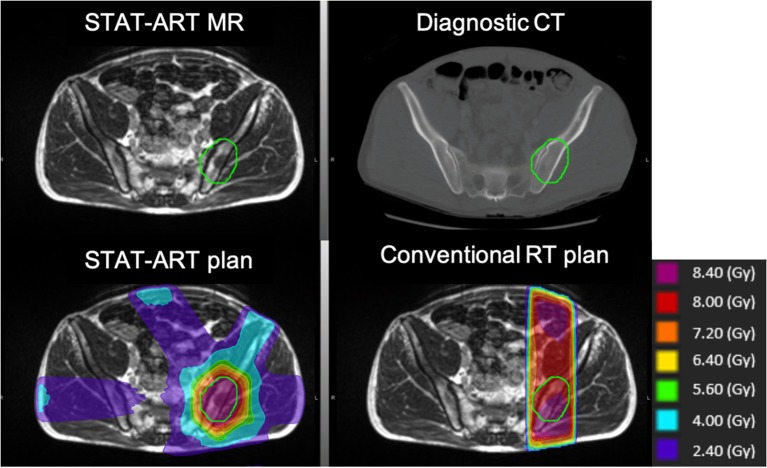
Clinical STAT-ART case of a pelvic bony metastasis treated to 8 Gy in a single fraction to the planning target volume in green. Comparison of diagnostic CT used for pre-planning (top right) and improved gross disease visualization on the TrueFISP treatment planning MR obtained using the MRIdian (top left). The resulting dose distribution for STAT-ART plan of a six-beam cobalt-60 dose distribution (bottom left) in comparison to a conventional plan with 10 MV AP/PA beams (bottom right). The STAT-ART plan shows a marked reduction of the high dose volume, particularly in anterior regions of normal tissues.
